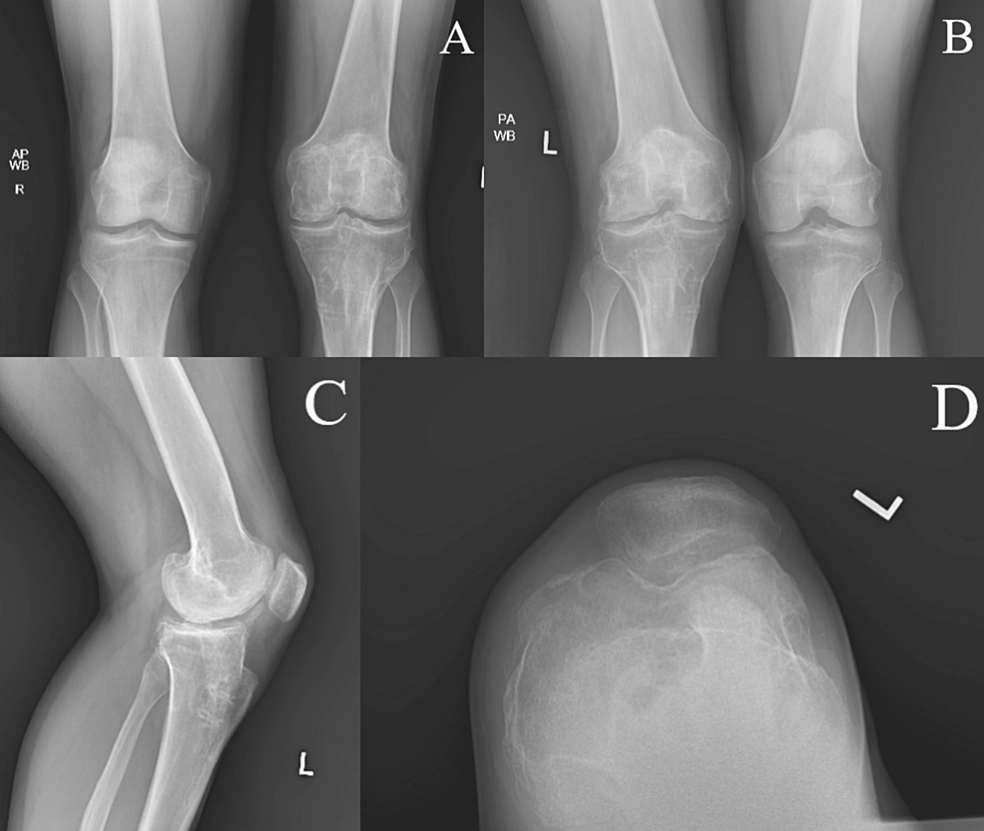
Anterior-posterior (A), posterior-anterior (B), lateral (C), and sunrise (D) radiographs of the patient's left knee one year post arthroscopic removal of loose bodies with lysis of adhesions, partial synovectomy, and hardware removal. Radiographs were also 18 years post autologous chondrocyte implantation. AP and PA showed a preserved joint space with mild subchondral irregularity.
